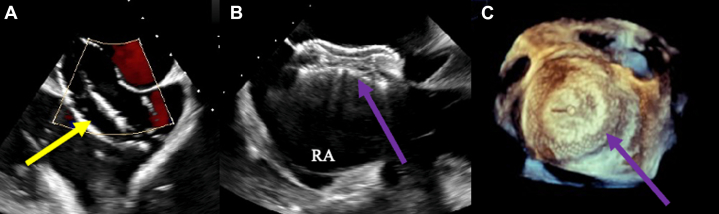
Assessment of the Unified Defect and Confirmation of Septal Occluder Position This figure demonstrates evaluation of the unified defect created after tendon transection and confirmation of final device position. (A) The unified defect is measured using a sizing balloon (yellow arrow) to determine the stretched diameter and guide device selection. (B) TEE confirms appropriate positioning of the septal occluder (purple arrow) and is used to verify that the divided septal tendon is incorporated within the device waist. (C) Three-dimensional TEE provides an en face view of the occluder (purple arrow), showing complete coverage of the defect and stable integration within the septal anatomy. RA = right atrium; TEE = transesophageal echocardiography.•An 8-F Amplatzer Trevisio delivery system was advanced via the 12-F femoral sheath. A 30-mm Amplatzer Septal Occluder was deployed across the defect incorporating the free portion of the divided tendon ridge. TEE confirmed that all rims of the defect were adequately captured (Figures 4B and 4C). A push-pull test confirmed device stability and exclusion of residual shunt.•At the conclusion of the procedure, all catheters and sheaths were removed, and hemostasis was achieved using a Z-suture at the femoral access site.
